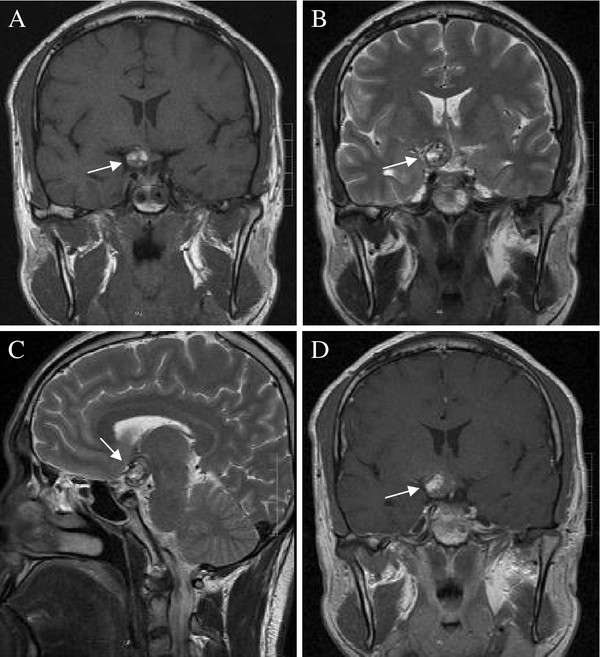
Magnetic resonance images of the cavernous malformation of the optic chiasm (arrows). (A) The mass lesion demonstrated diffuse hyperintensity on T1-weighted imaging and mixed signal intensity; (B-C) The lesion was surrounded by a marked hypointense peripheral rim of hemosiderin on T2-weighted imaging. (D) No significant contrast enhancement was observed after the enhanced scanning.
